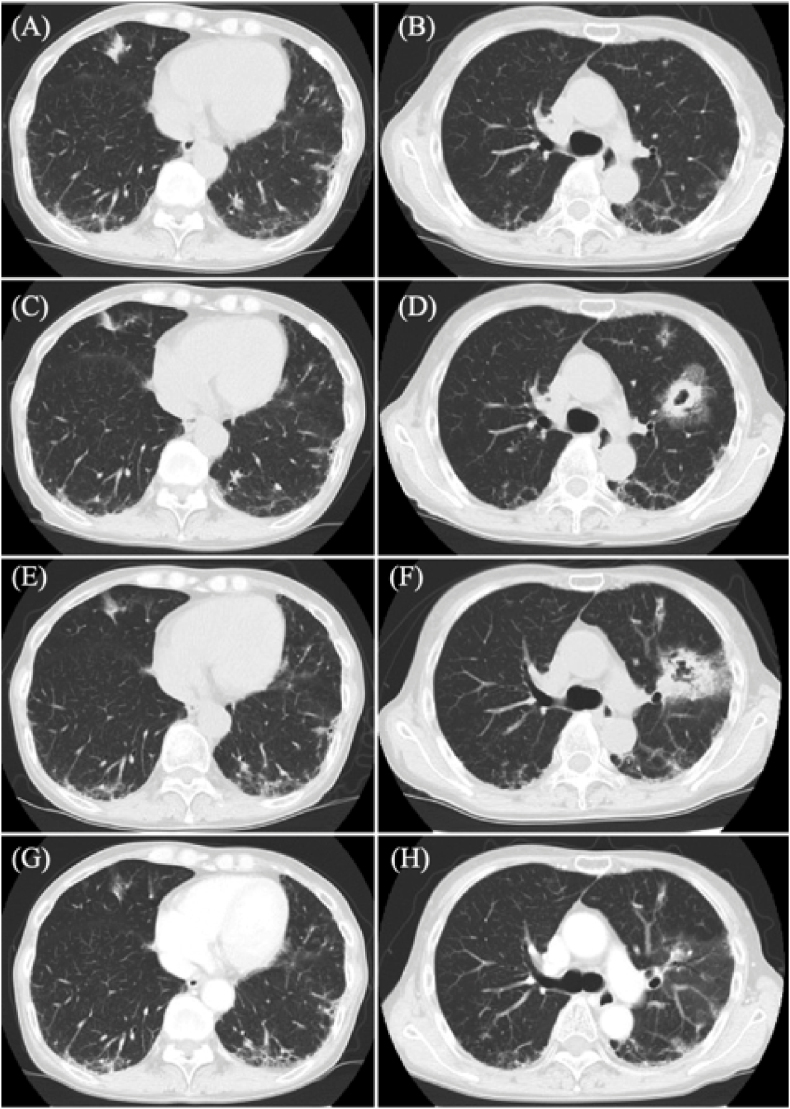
(A–B) Chest computed tomography (CT) revealed a nodular shadow at the base of the right lung five months after the diagnosis of anti-MDA5 antibody-positive dermatomyositis (day 0). (C–D) Three weeks after starting voriconazole (day 22), CT showed a reduction in the size of the known lesion in the right middle lobe, but a new cavitary shadow appeared in the left upper lobe. (E–F) CT after initiating ampicillin-sulbactam and amphotericin B (day 48) showed worsening of the cavitary shadow in the left upper lobe. (G–H) CT one month after the addition of terbinafine (day 65) showed improvement in the cavitary shadow in the left upper lobe.
